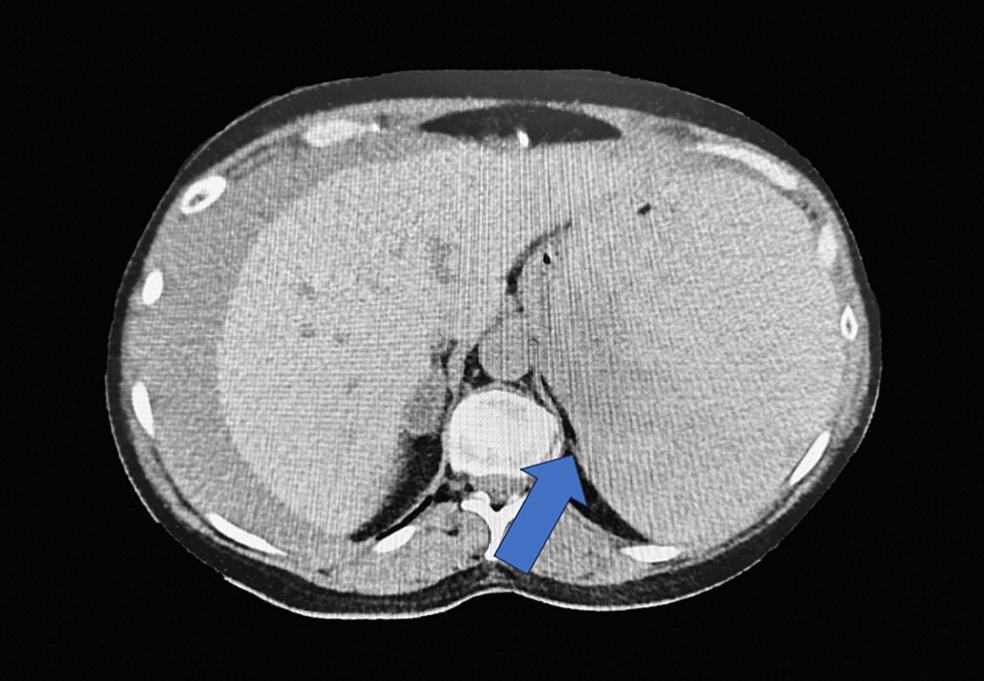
Computed tomography whole abdomen Arrow: cross-sectional view of the abdomen. Spleen not visualized secondary to large perisplenic hematoma. The sentinel hematoma in the left upper quadrant measures 13 cm × 17 cm.
